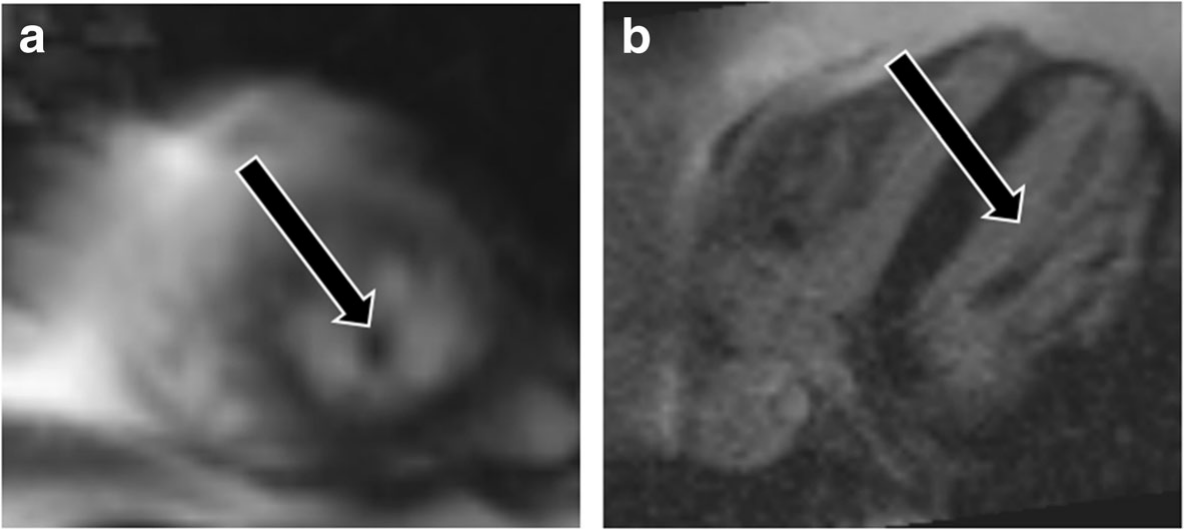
Accessory papillary muscle mimicking thrombus. a Short-axis SSFP shows hypointense region in the ventricular apex (arrow), thought to be thrombus on echocardiography. b Delayed-enhanced images with an inversion time > 600 ms show that the structure is not hypointense (arrow), consistent with an accessory papillary muscle rather than thrombus
